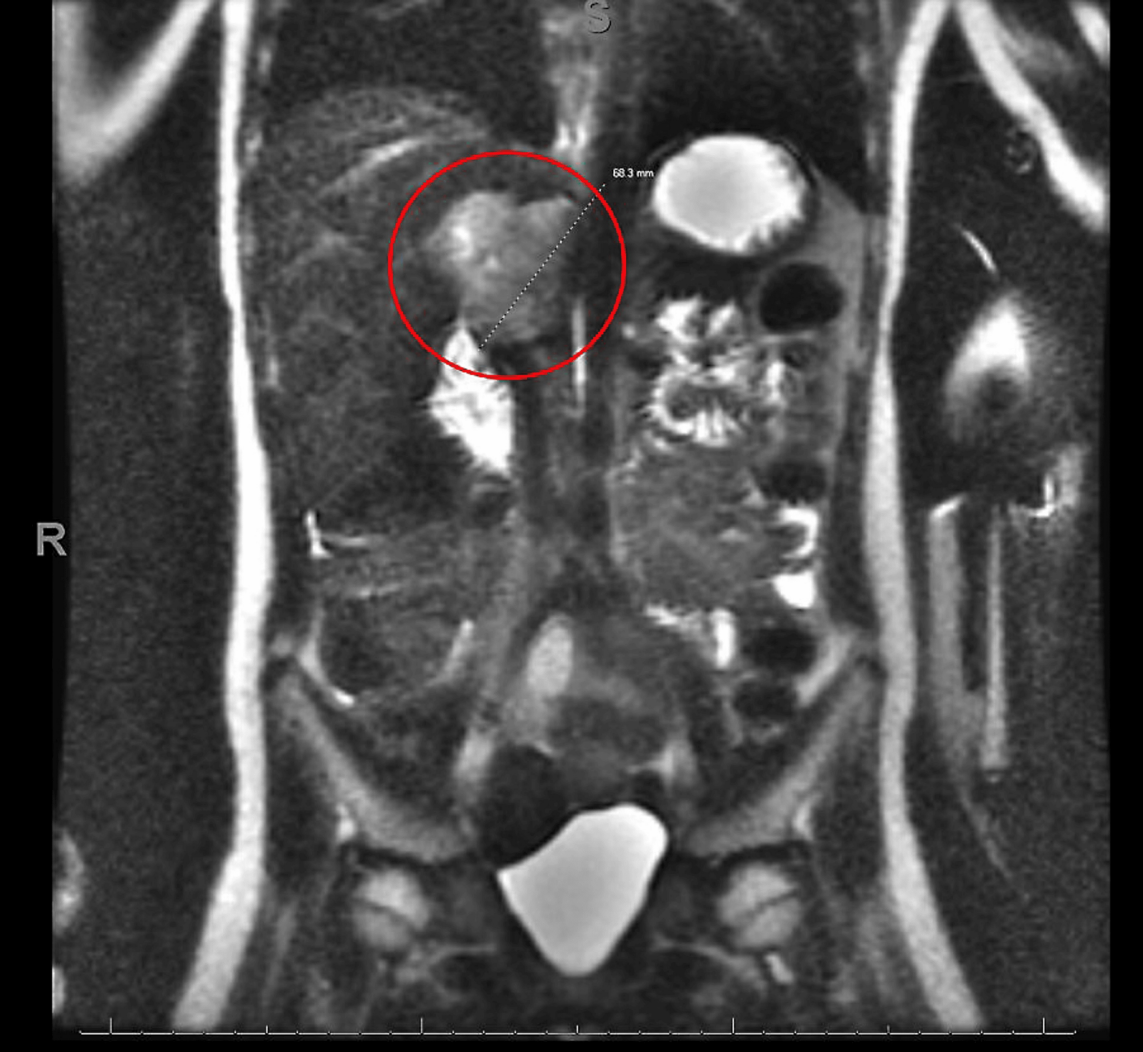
Coronal plane MRI view of the patient’s abdomen. The mass is highlighted by a red circle.
